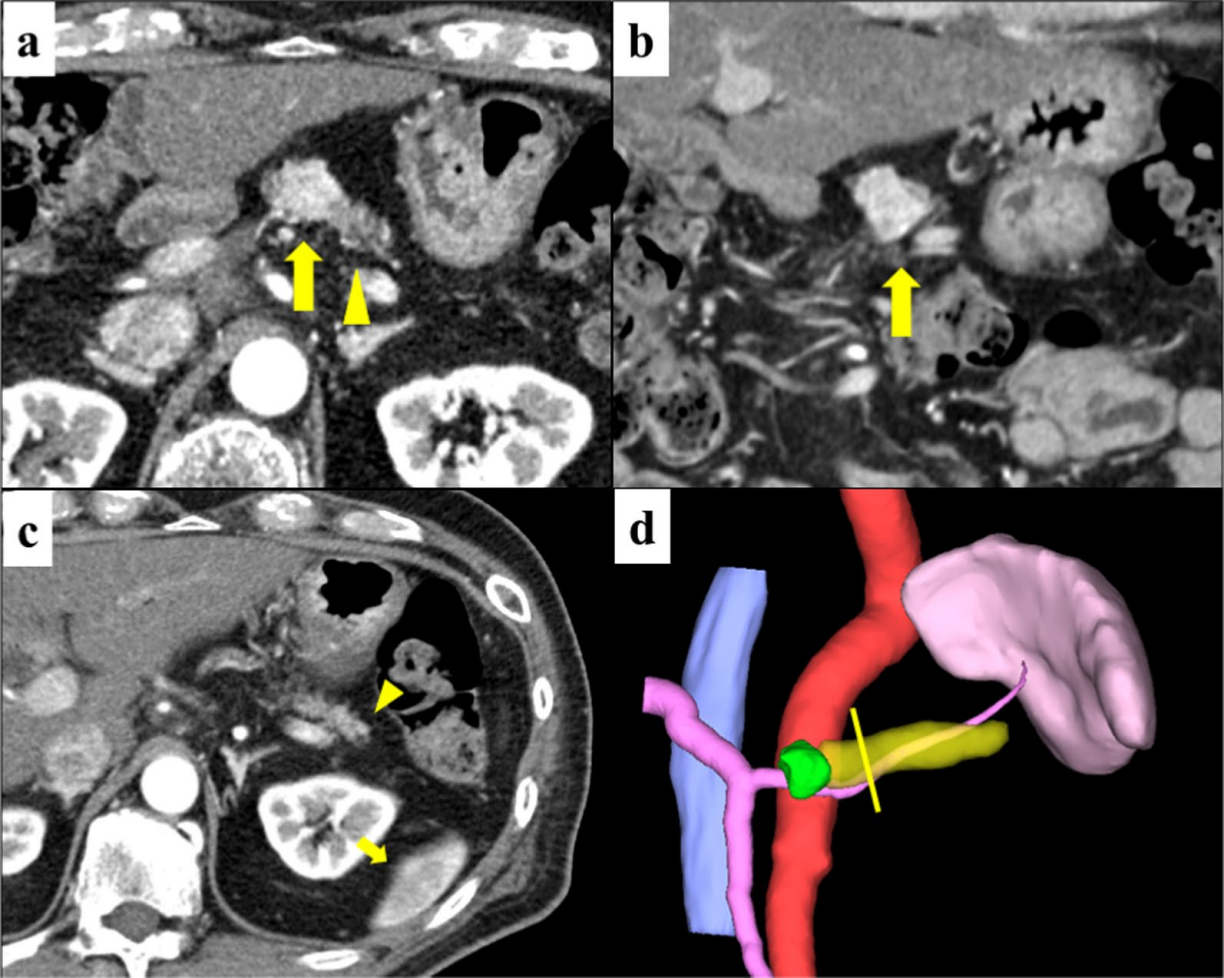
Contrast-enhanced abdominal computed tomography scan. a Axial view: The 19-mm tumor with contrast enhancement (arrow) is located at the pancreatic body near the pancreatojejunostomy site. The remnant pancreas is atrophic (arrowhead). b Coronal view. c Association between the distal edge of the pancreas (arrow) and the spleen (arrowhead). d 3D construction image (Synapse Vincent; green, tumor; yellow, remnant pancreas; yellow line, estimated transection line)
